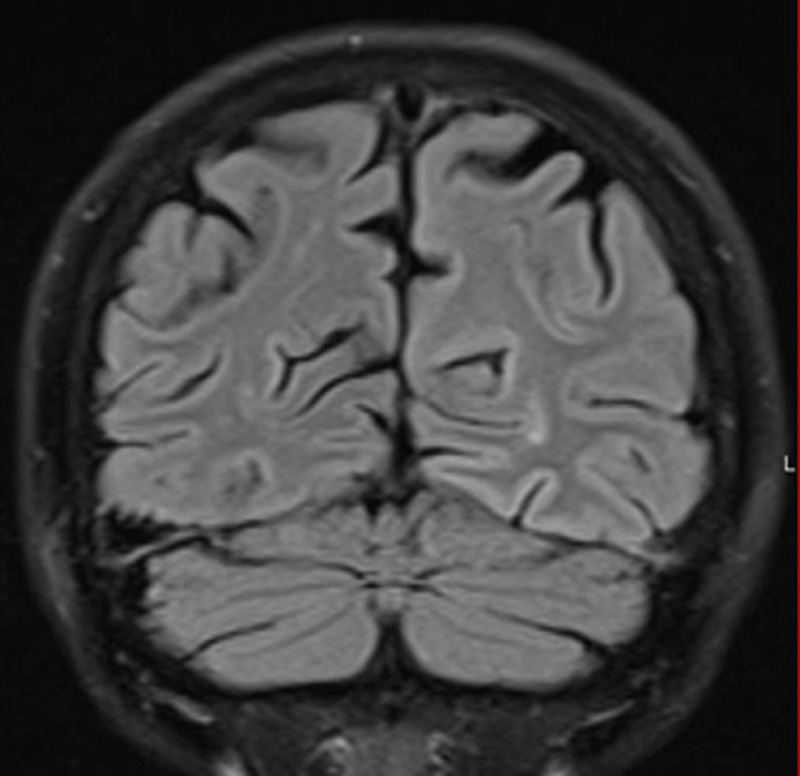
At the time of MRI, 14-3-3 protein, a characteristic and suggestive biomarker for Creutzfeldt Jakob disease, was negative. Fifteen days later a second analysis was positive.
